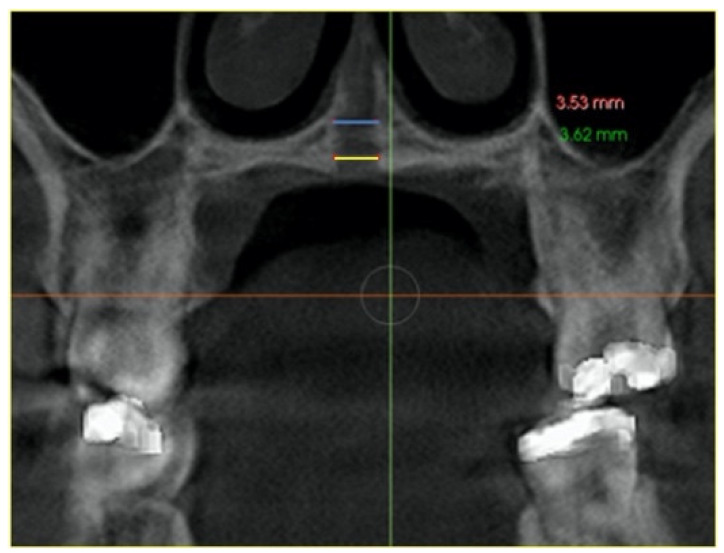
Table 4 jcm-11-04652-t004: Radiographic CBCT measurements to quantify skeletal, dentoalveolar and dental expansion. Sutural expansion, intermolar width and palatal maxillary width (SEM, IMW and PMW) were measured at first molar and quantified on the same coronal cross-sectional slice.
